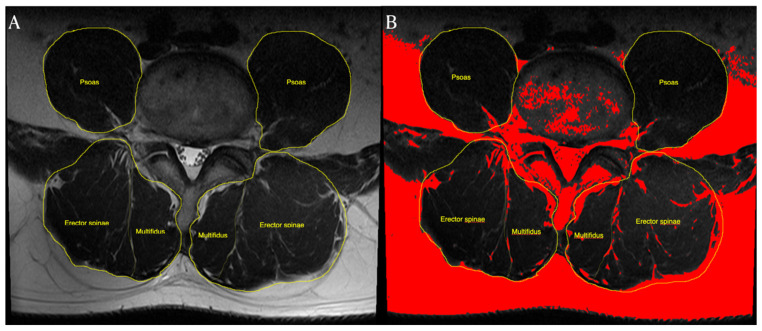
We defined the back muscles as consisting of the multifidi and erector spinae muscles. This region was manually traced. The CSA of the back muscles and multifidi were measured separately: (A) the rate of fat infiltration was measured using the pseudocoloring technique in the software. Fat tissue on axial T2-weighted MR image was converted to red. We calculated the portion of red area among the CSA of back muscles (B).
